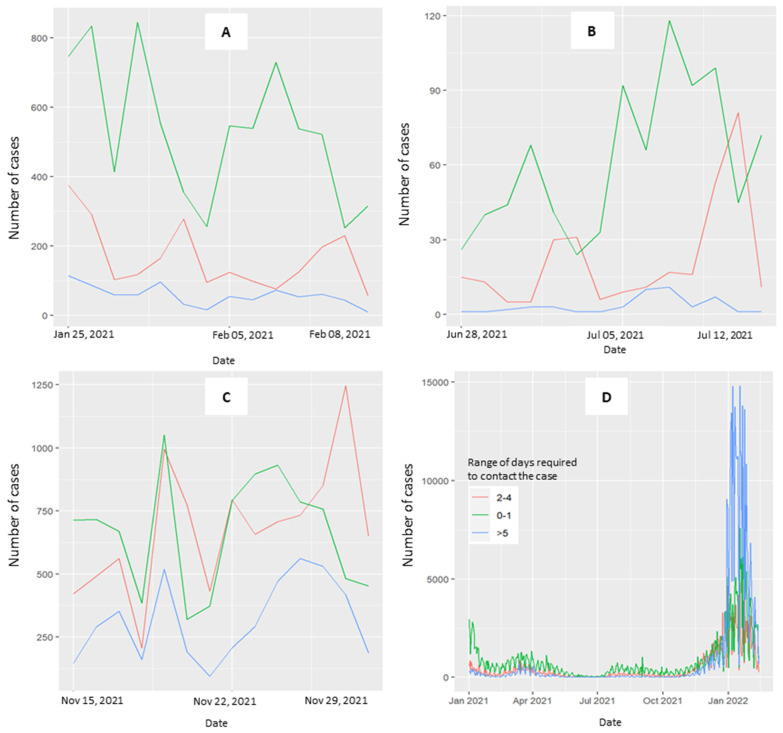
Trend of the range of days required to perform the CT activity. Patients testing positive for COVID-19 were contacted by the health prevention department at 0–1 days (green line), 2–4 days (red line), and 5 days (blue line) after the diagnosis of positivity. (A) Period with a decreasing curve, not approaching zero, from 25 January–8 February 2021. (B) Period where the number of cases with stable curves is at zero for 15 days, from 28 June–12 July 2021. (C) Period with a rising curve, from 15–29 November 2021. (D) Overall period.
